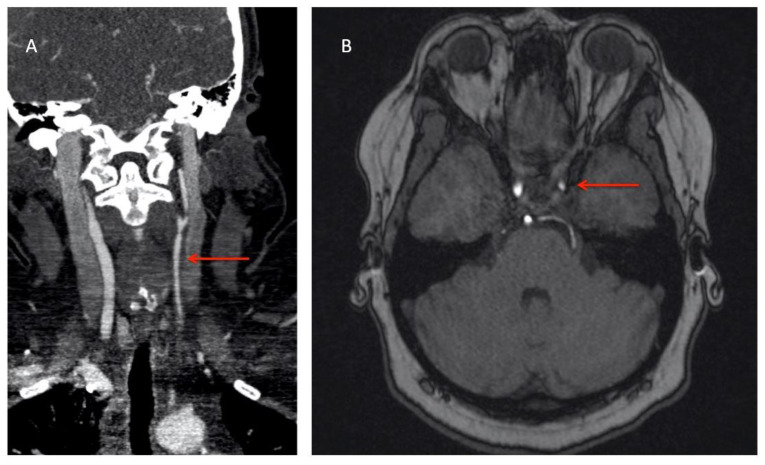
(A) CT-A demonstrating extensive, diffuse narrowing of the left common carotid artery and extracranial internal carotid artery; (B) MR brain with and without contrast showing left internal carotid artery terminus narrowing.
